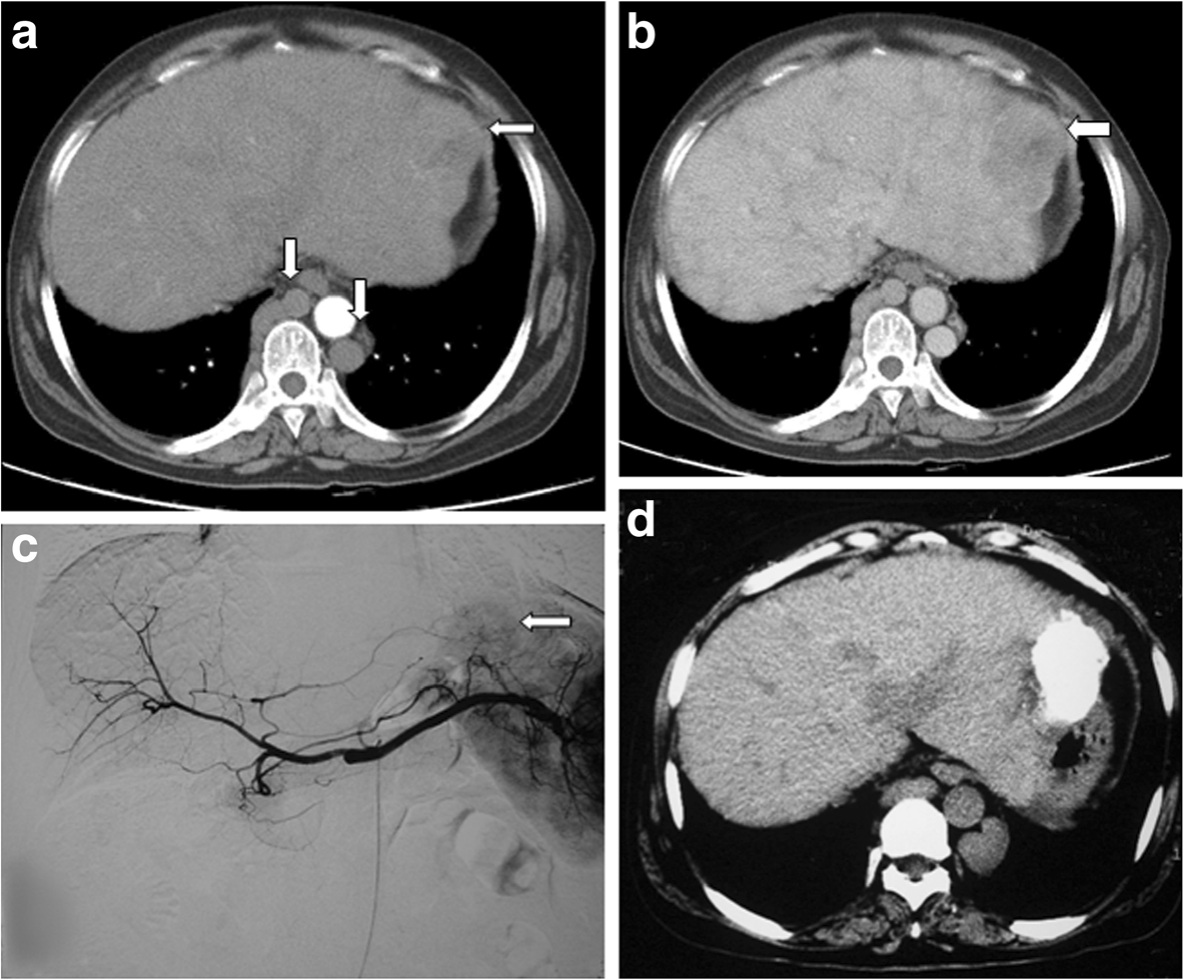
51-year-old woman with HCC in BCS. (a) Contrast-enhanced transverse CT scan showing a large mild heterogeneous enhanced nodule(⇦) in the left liver during arterial phase and obvious cirsoid azygous vein and semi-azygous vein (⇩) and liver congestive cirrhosis. (b) Washout of the nodule on portal venous phase (⇦). (c) Angiography showing heterogeneous and contorted tumorous veins in the left liver (⇦) during arterial phase. TACE was given at the first time. (d) Contrast-enhanced transverse CT scan showing that the nodule was obtained stable effect after 2 TACE treatments for 14 months. Lipiodol was located equably in the nodule.
